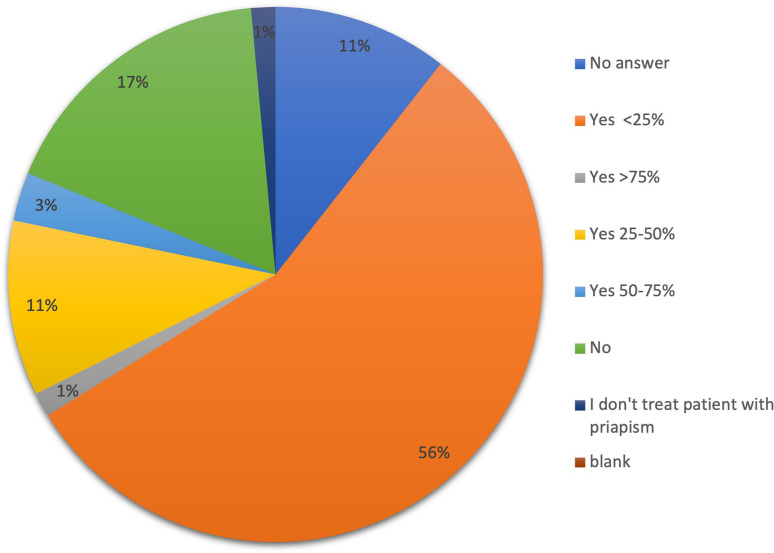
Use of penile magnetic resonance imaging in the evaluation of a patient with priapism.
